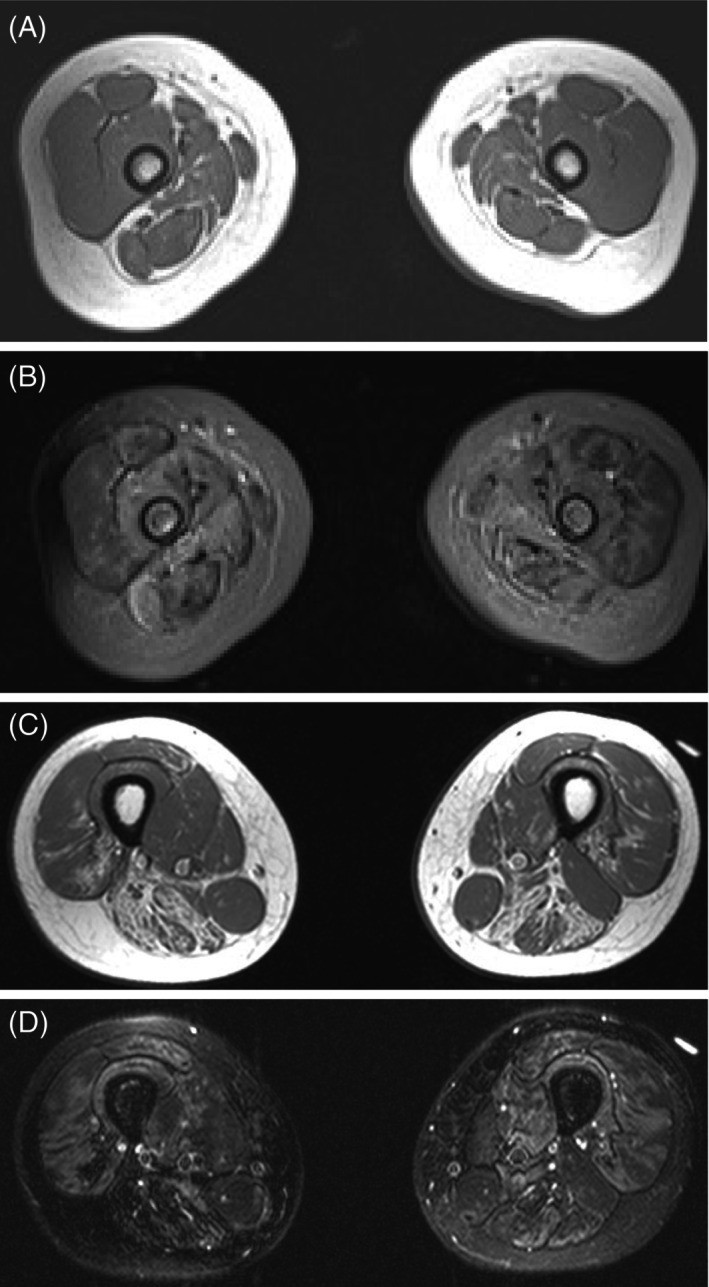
Representative T1‐weighted and STIR muscle MRI showing fatty infiltration and edema. Axial thigh MRI images of patients P6 and P8. (A) T1‐weighted image of P6. (B) STIR at same level as (A). (C) T1‐weighted image of P8. (D) STIR at same level as (C).
